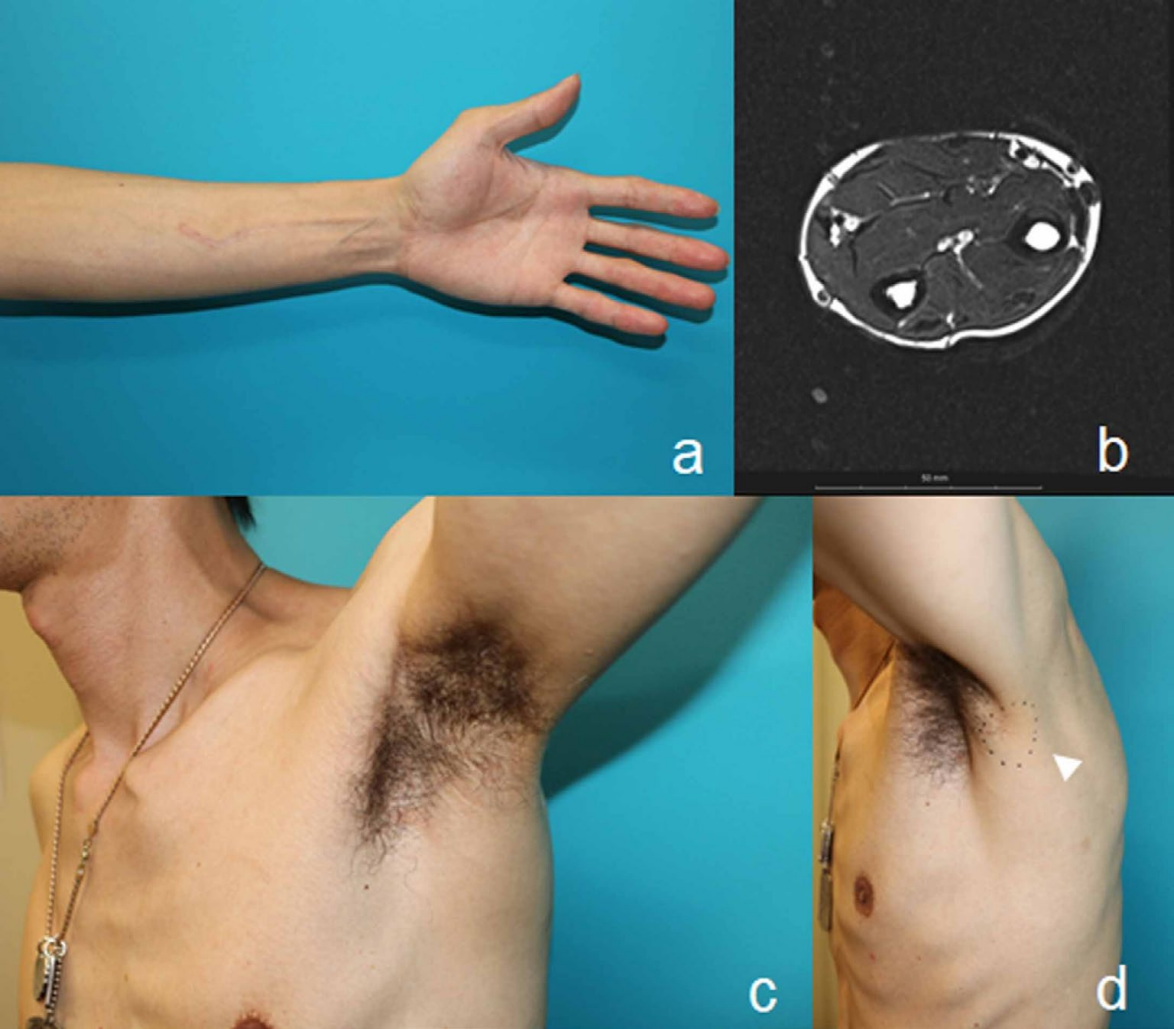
The forearm where the schwannoma was removed and the donor site, 13 months after surgery. (a) Postoperative hypesthesia of the index and middle fingers gradually improved. (b) Magnetic resonance imaging shows no recurrence of the tumor. (c) Scarring at the donor site is acceptable. (d) The site of the postoperative hypesthesia of the donor site is gradually reduced in size, leaving a 3 × 4 cm area of hypoesthesia behind the axilla (arrowhead).
